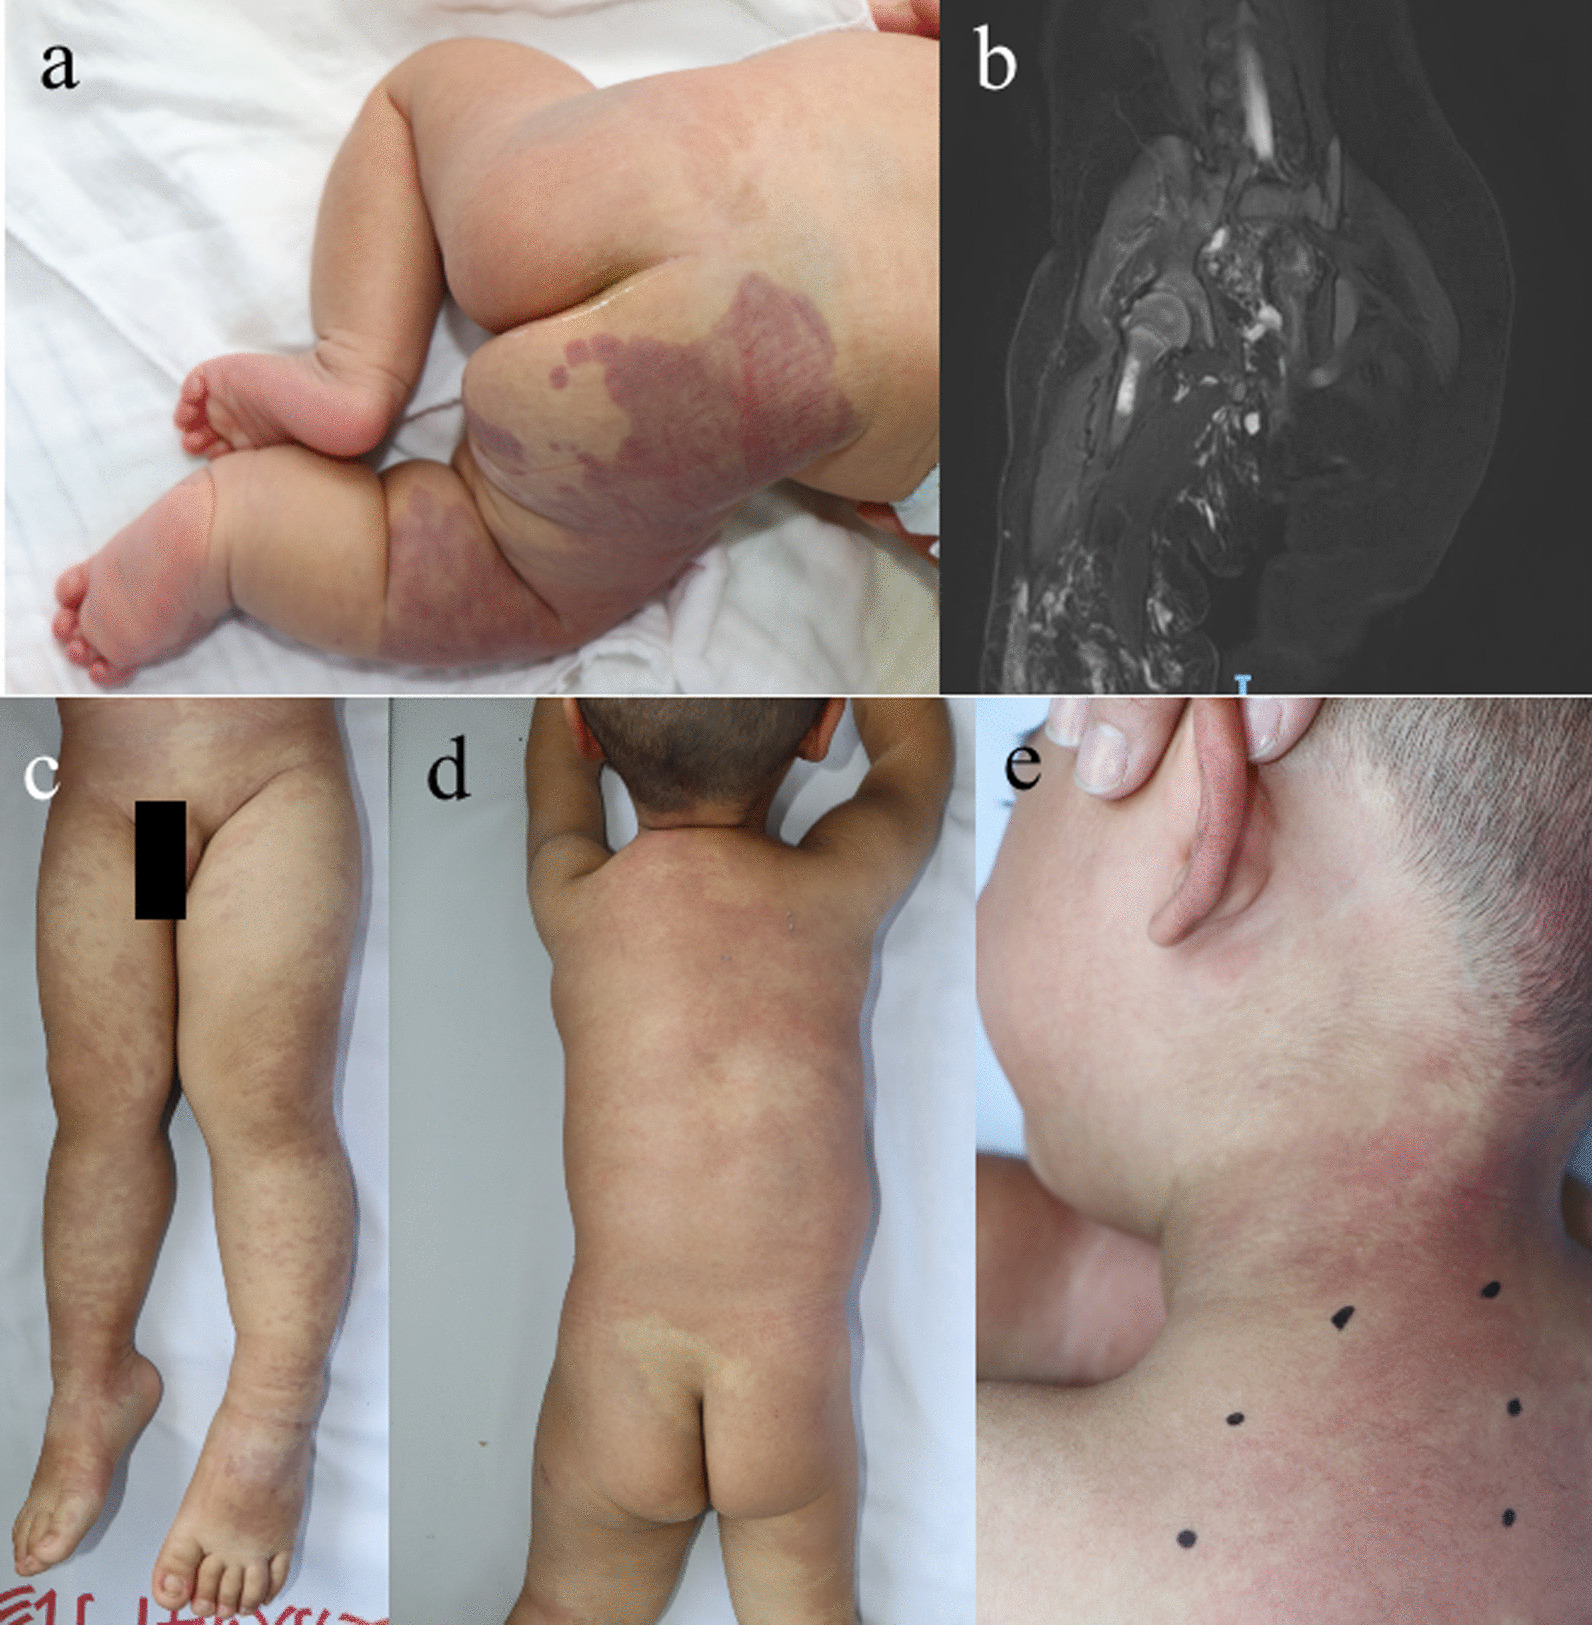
Pediatric PROS patients harboring PIK3CA somatic non-hotspot mutations. a Patient P53, a 1-month-old girl with KTS and PIK3CA c.328_330delGAA;p.E110del mutation. She had right lower limb and foot overgrowth. b T2 phase of MRI showed multiple streaky vascular shadows with hypersignal pressure on subcutaneous soft tissue of buttock and proximal femur. c Patient P83, a 20-month-old boy with CLOVES and PIK3CA c.2176G > A;p.E726K mutation. He had left lower limb overgrowth, d subcutaneous mass on the left back, e and epidermal nevi on the neck
